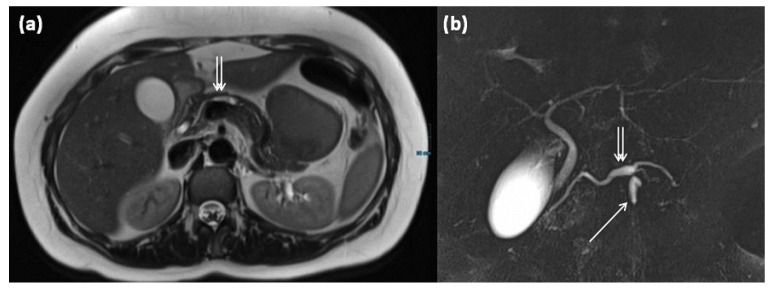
MRI of a 39-year-old female patient with T2w (a) and magnetic resonance cholangiopancreaticography (MRCP) (b). Images show a clear demarcated main duct dilatation in terms of a main duct IPMN (double arrow). Furthermore, the extension of a branch duct (arrow) can be seen, thus of a combined main and branch duct IPMN is present (mixed-type).
